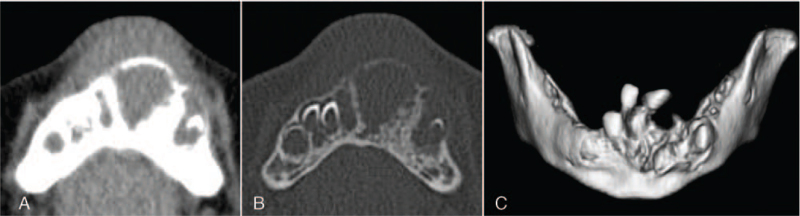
Axial CT image showing a large mass in the mandibular alveolus. Soft tissue window. Bone window. 3D reconstruction of CT image showing numerous tooth germ displacements.
